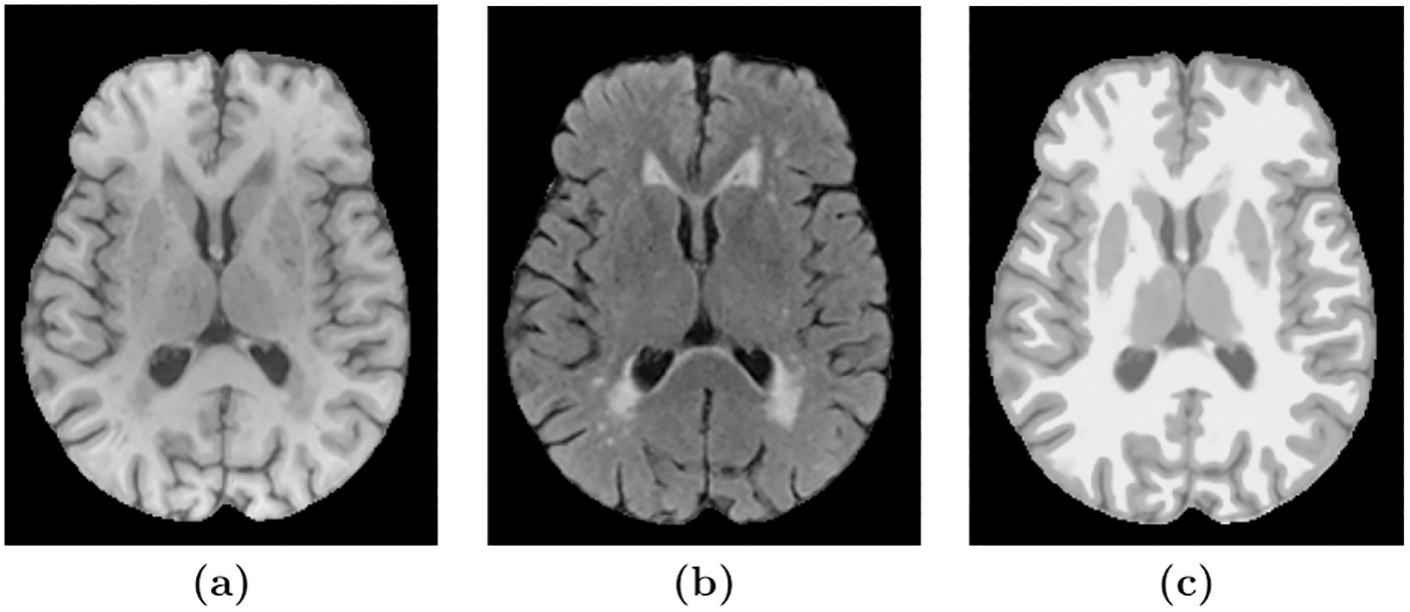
The MRI sequences vs. the standardized image. Images (a) and (b) show T1-w and a FLAIR images of a subject, respectively, and image (c) shows a standardized image made of SegAE segmentations, which is free of inhomogeneity artifacts and WMHs.
